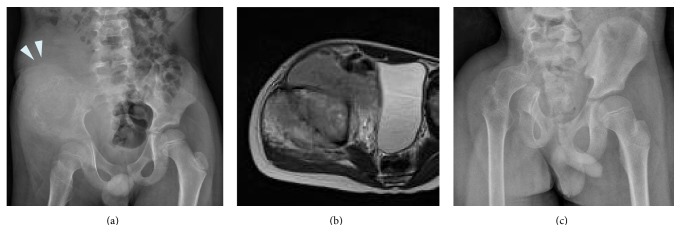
(a) Preoperative anteroposterior radiograph of the pelvis of an 8-year-old boy who had Ewing sarcoma in the ilium and acetabulum. (b) Axial T2-weighted MR image showing the tumor arising in the acetabulum. (c) Postoperative radiograph one year after hip transposition.
